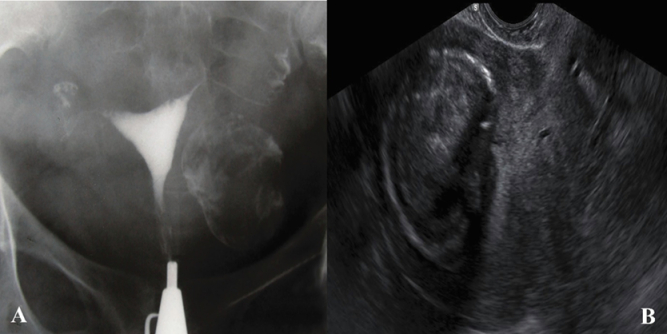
A) HSG of a 35-yr-old woman with primary infertility. The uterine cavity and fallopian tubes have a normal view. A calcified area is seen on the left side of the uterine cavity. B) The fibroma was approved by a 2-D ultrasound examination.
